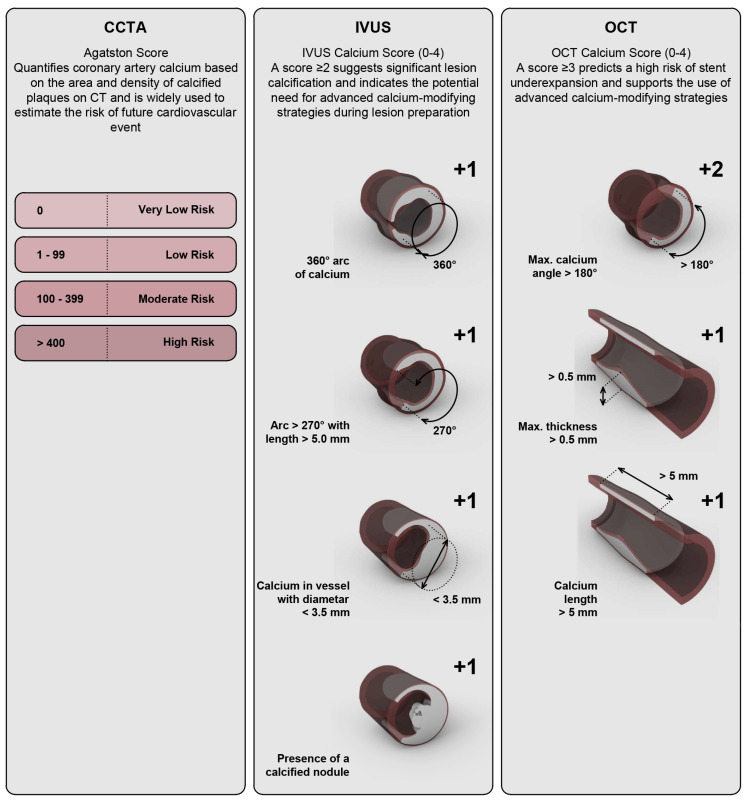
Coronary artery calcium scores derived from different imaging modalities. CCTA—coronary CT angiography; IVUS—intravascular ultrasound; OCT—optical coherence tomography.
